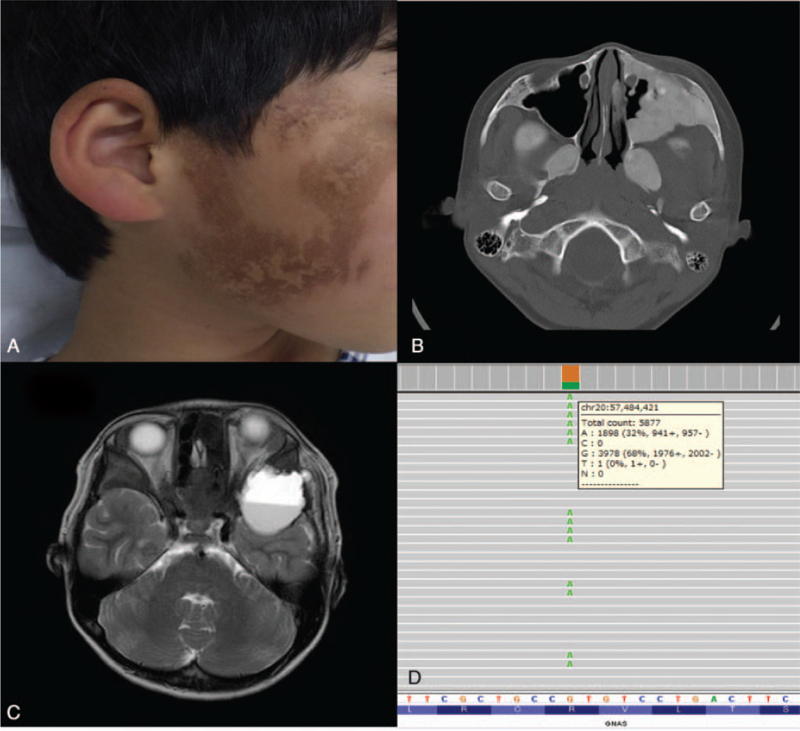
Dermatological findings and radiological examination of a patient with fibrous dysplasia and aneurysmal bone cyst before treatment and genetic analysis. (A) Café-au-lait macules from birth on the right-hand side of the patient's face were identified. (B) CT revealed expansile ground-glass opacity on his bilateral pterygoid process and on the posterior wall of the left maxillary sinus (axial CT bone window of the skull base showed ground-glass opacification on his bilateral pterygoid processes and on the anterior and posterior bony walls of the maxillary sinus) and we diagnosed fibrous dysplasia. (C) Transverse T2-weighted MRI showed the cystic bone tumor with fluid-fluid level in the left middle cranial fossa with left optic nerve compression, indicating secondary ABC. (D) Genetic analysis by next-generation sequencing showed a somatic activating mutation in *GNAS* at c.602G > A (p.R201H) at a variant allele frequency of 32.3%. ABC = aneurysmal bone cyst, CT = computed tomography, MRI = magnetic resonance imaging.
